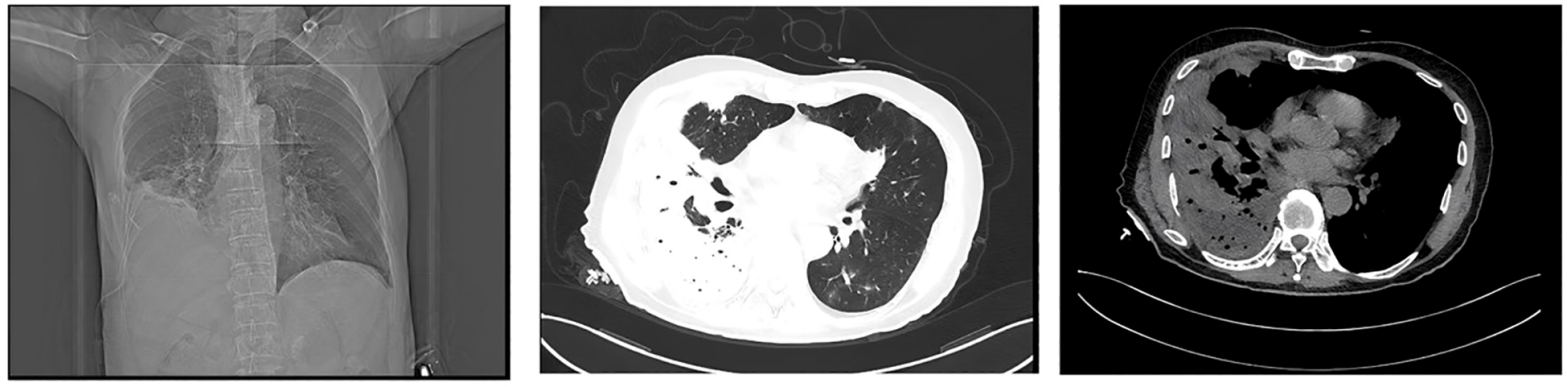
On post-procedure day 3, a follow-up chest CT demonstrated a significant reduction in abscess cavity fluid accumulation.
